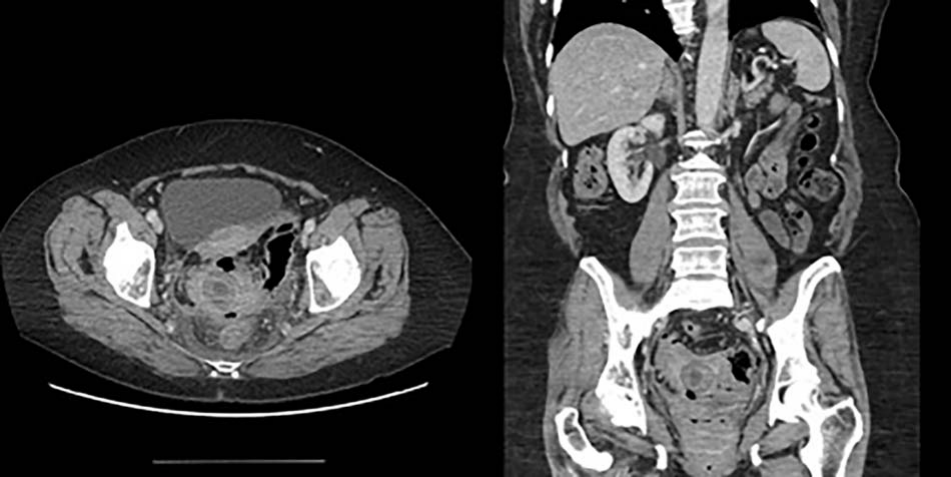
Patient 2 representative CT scan cuts.
